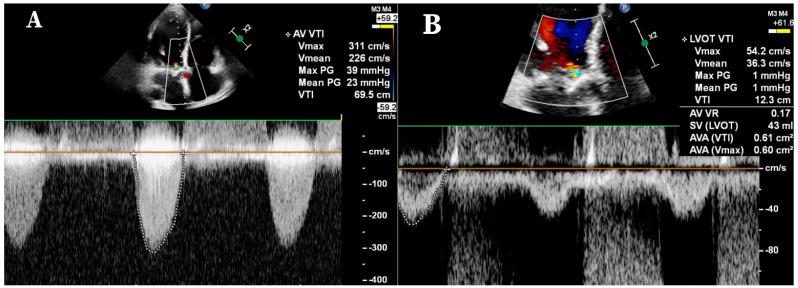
Low-flow, low-gradient aortic stenosis detected by transthoracic echocardiography. Continuous wave Doppler imaging shows low gradients and flow velocity across the calcified aortic valve. Panel (A): mean and peak gradient at 23 mmHg and 39 mmHg, respectively; maximum velocity at 3.11 m/s—non-significant values presented. Panel (B): left ventricular outflow tract velocity time integral is 12.3 cm; calculated aortic valve area is 0.61 cm^2^—significant and critical valve disease observed. AV: aortic valve; Vmax: maximum velocity; Vmean: mean velocity; VTI: velocity time integral; PG: pressure gradient; LVOT: left ventricular outflow gradient; VR: velocity ratio; SV: stroke volume; AVA: aortic valve area.
